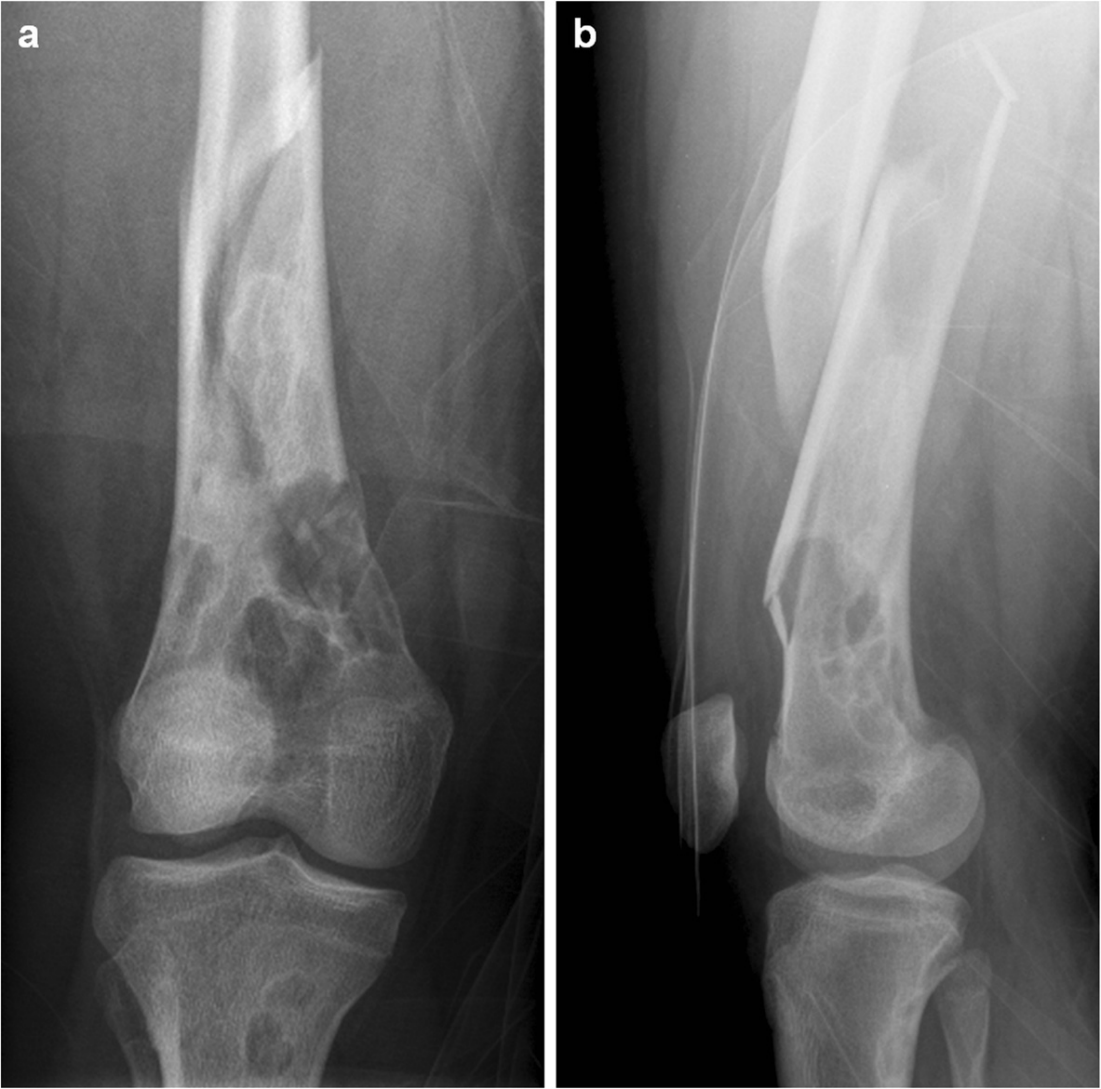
Anteroposterior (a) and lateral (b) radiographs displaying a pathological comminuted spiroid fracture of the right distal femoral diaphysis originating from a cluster of multiple large NOFs.
